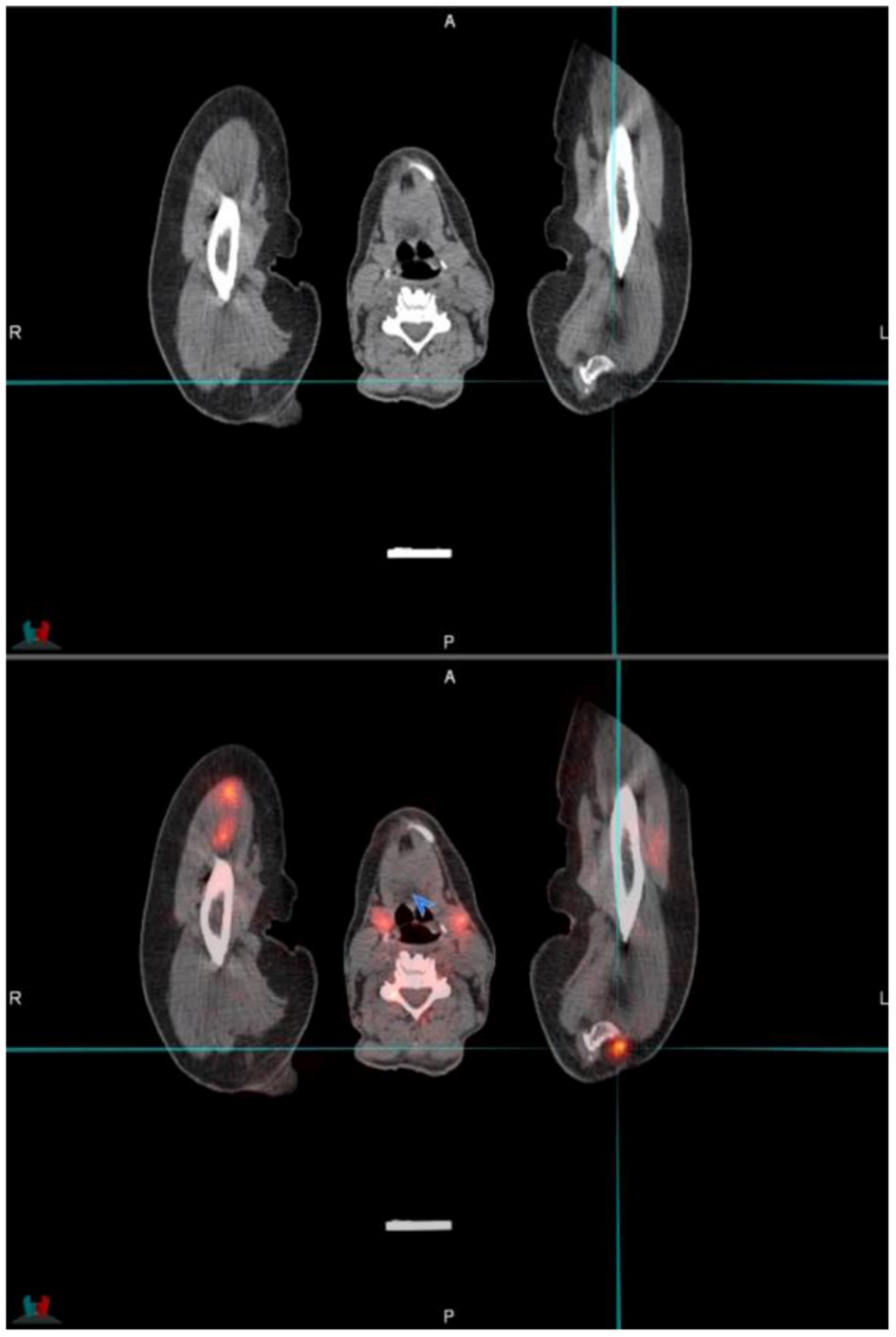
Transaxial CT and fused PET/CT images through the upper limbs and cervical area. The cross-hairs indicated a focus of activity that is misregistered due to patient movement of the arms—the activity should be located in the coracoid process of the left scapula that is in close proximity.
